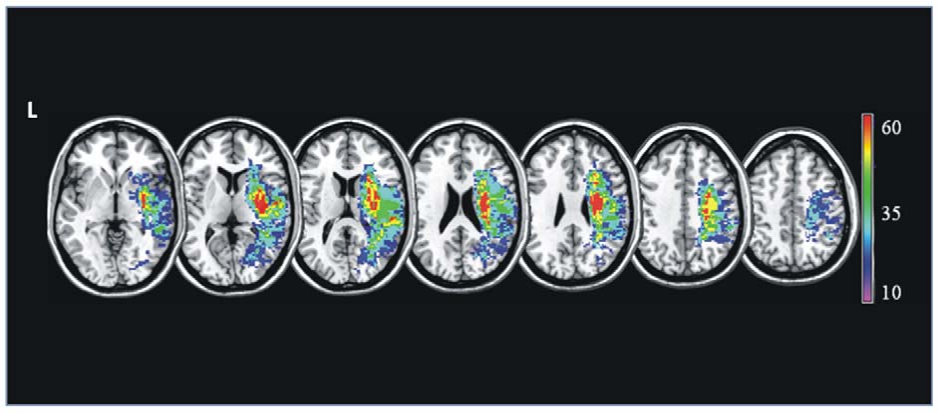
Lesion distribution. The damage evident in MRI images was reconstructed for each studied patient and plotted using MRIcro software. A T1-weighted template comprising 12 axial slices was used to demarcate lesions for every patient. The colour scale indicates the percentage of overlapping lesions across patients.
